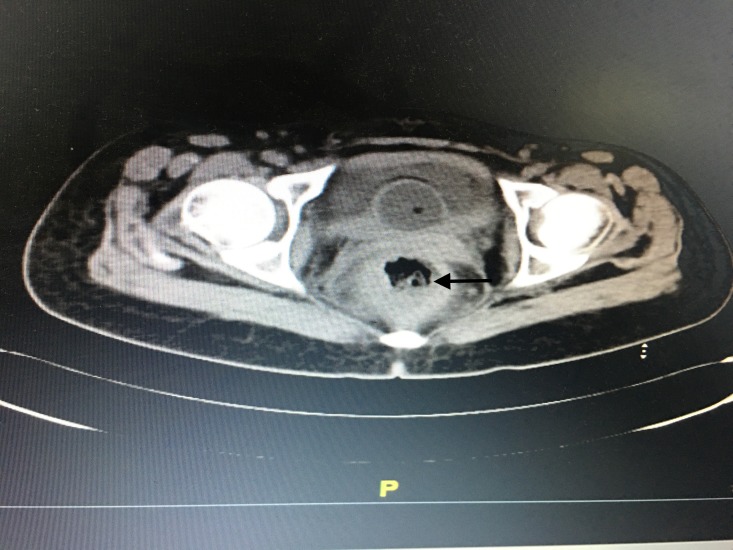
Computed tomography picture showing inflamed anal canal wall with sloughed out mucosa (arrow).
